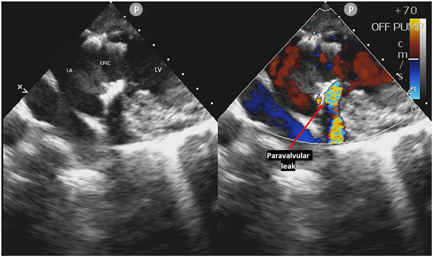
Paravalvular leak in anterolateral trigone of Epic mitral bioprostheses (Video S1). Three of the four Epic mitral bioprostheses were noted to have a paravalvular leak at the anterolateral commissure of the mitral prothesis sewing cuff postcardiopulmonary bypass epicardial echocardiographic interrogation
